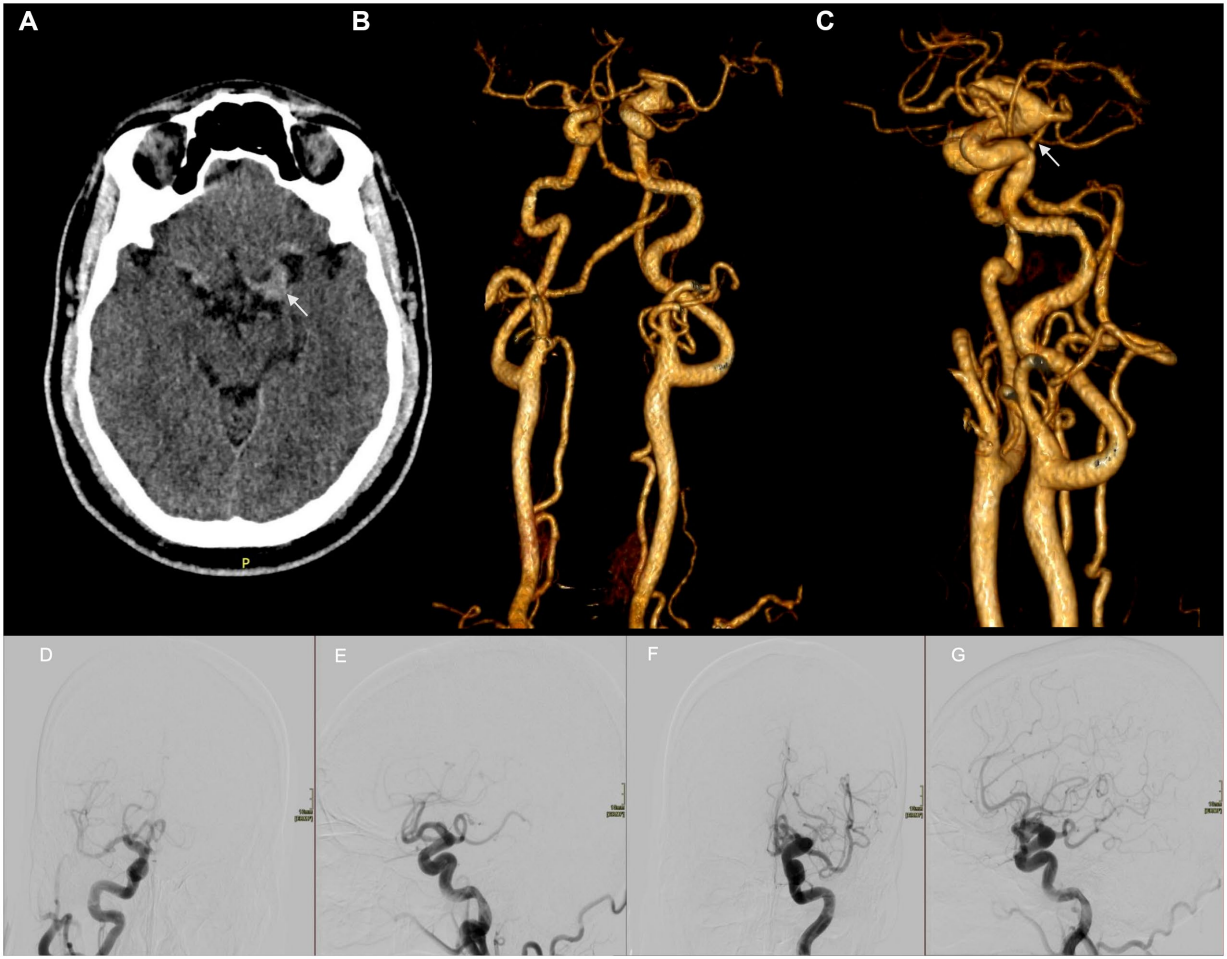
(A) An axial non-contrast CT scan shows a fusiform aneurysm of 10 mm diameter of the M1 segment of the left middle cerebral artery with increased density. (B) A three-dimensional (3D) reconstruction of CT angiography of the cerebral arteries shows a fusiform aneurysm involving the ophthalmic and posterior communicating segments of the left internal carotid artery. The course of both internal carotid arteries and the vertebrobasilar system is tortuous. (C) A lateral view of the 3D reconstruction of CT angiography of the cerebral arteries. Cerebral angiography. AP (D,F) and lateral projections (E,G) of internal carotids showing fusiform dilation and tortuosity of both internal carotid arteries from the petrous segment to the terminal segment. No saccular aneurysms are seen. (D,E) Right carotid. (F,G) Left carotid.
